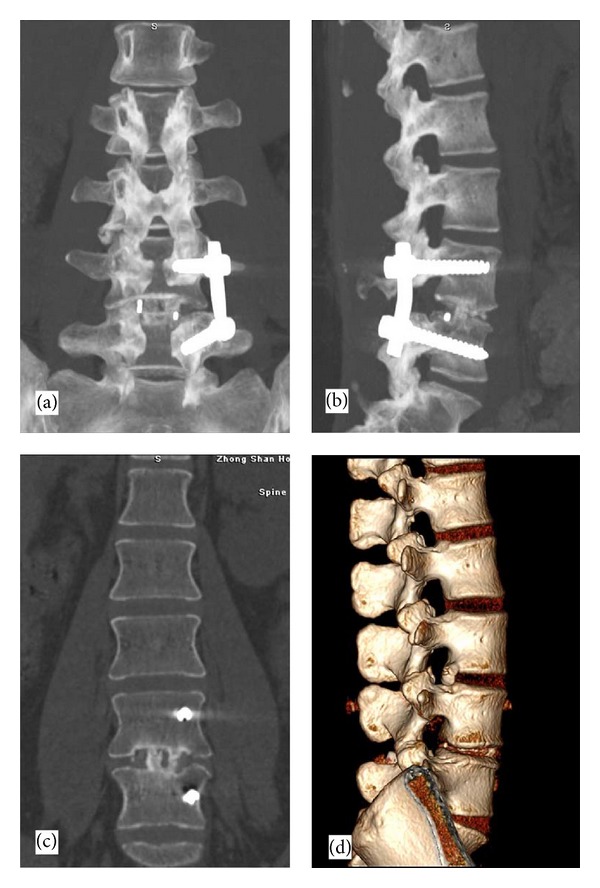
Follow-up three-dimensional CT scan revealing bony fusion at 12 months after TLIF. (a) Anteroposterior view; (b) lateral view; (c) coronal view; (d) lateral view with three-dimensional holographic reconstruction.
